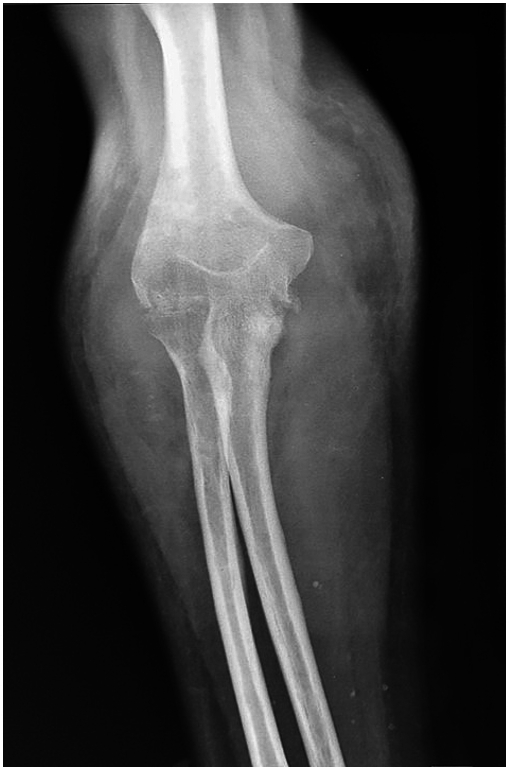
Anteroposterior radiograph of the right elbow showing marginal periarticular osteolysis and elbow subluxation.
